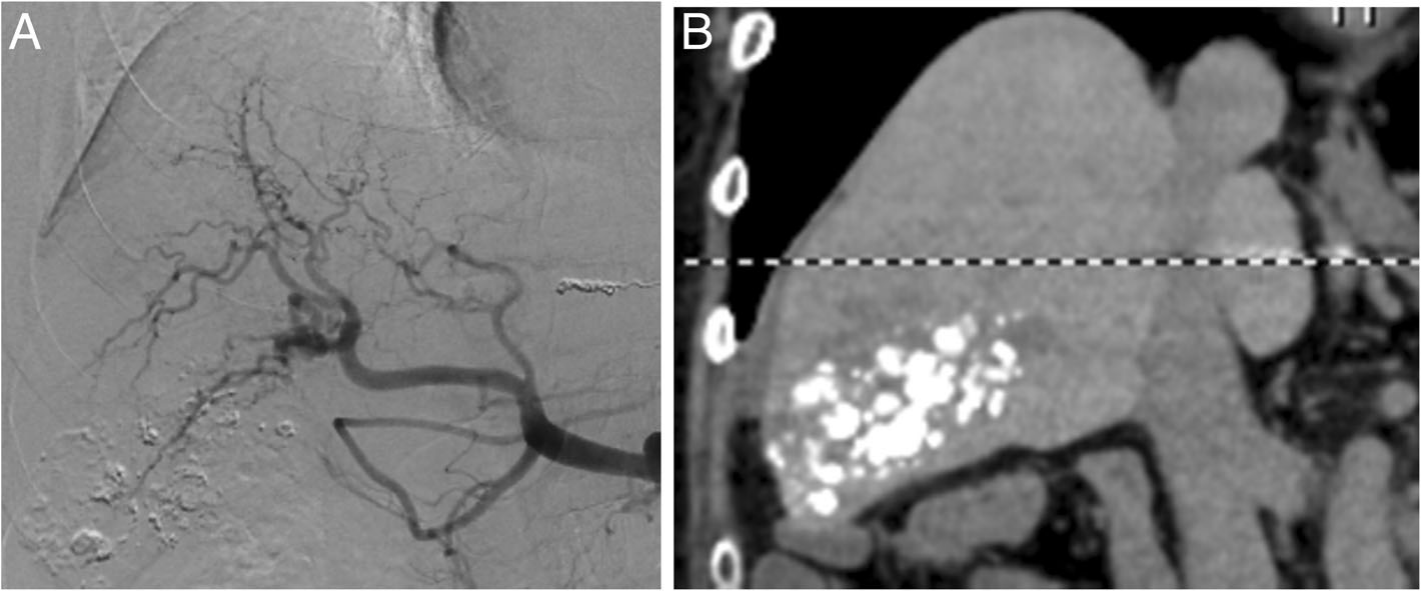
Diagnostic images at the fourth chemoembolization procedure. a Digital subtraction angiography. b Plain CT after chemoembolization. The main tumor is markedly diminished with no enhancement, and lipiodol showed spotty but strong accumulation
